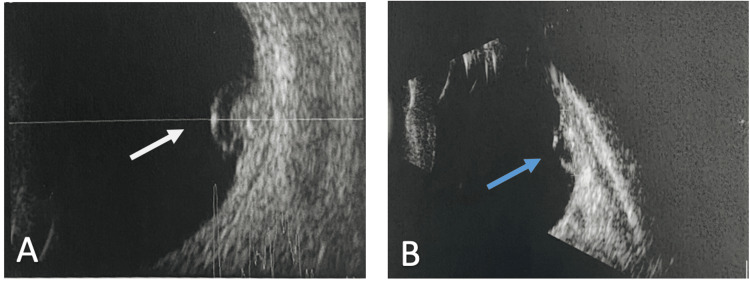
Ocular ultrasound of the right eye showing the retinocytoma (white arrow) at the time of presentation (A) and the mass two years (blue arrow) later (B).
